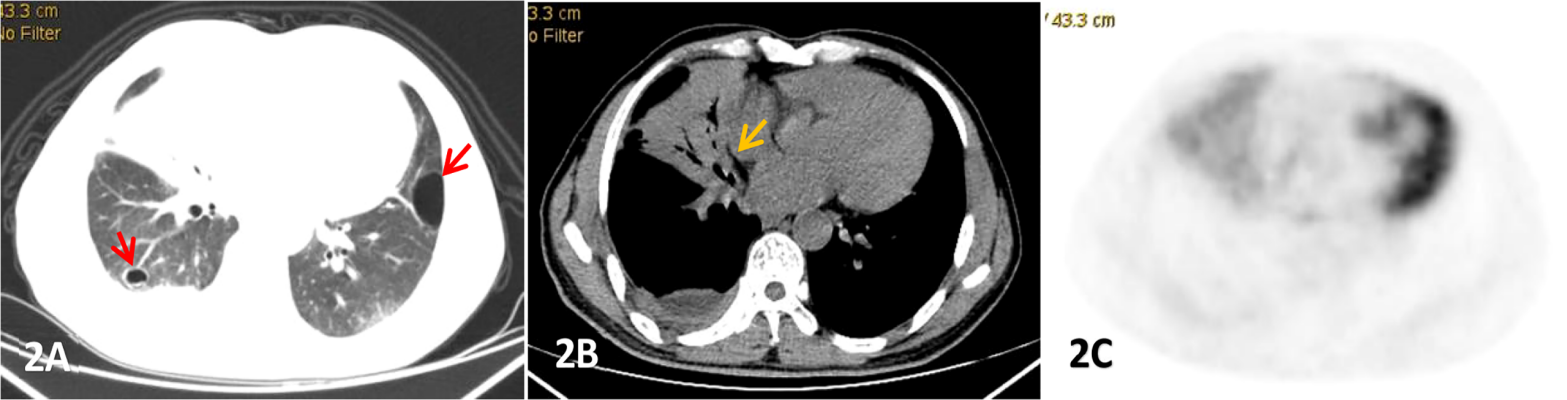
Male, 53 years old, cough and expectoration accompanied by right lower chest pain for more than 2 months, (A-C) were lung, mediastinum CT transection scan and PET transection image respectively,. Large consolidation shadow was showed in the middle lobe of the right lung with air bronchial signs(yellow arrow),which FDG uptake increased with an SUVmax of 3.83, right pleural thickening and pleural effusion, and cystic lesions(red arrow) were also observed in both.
